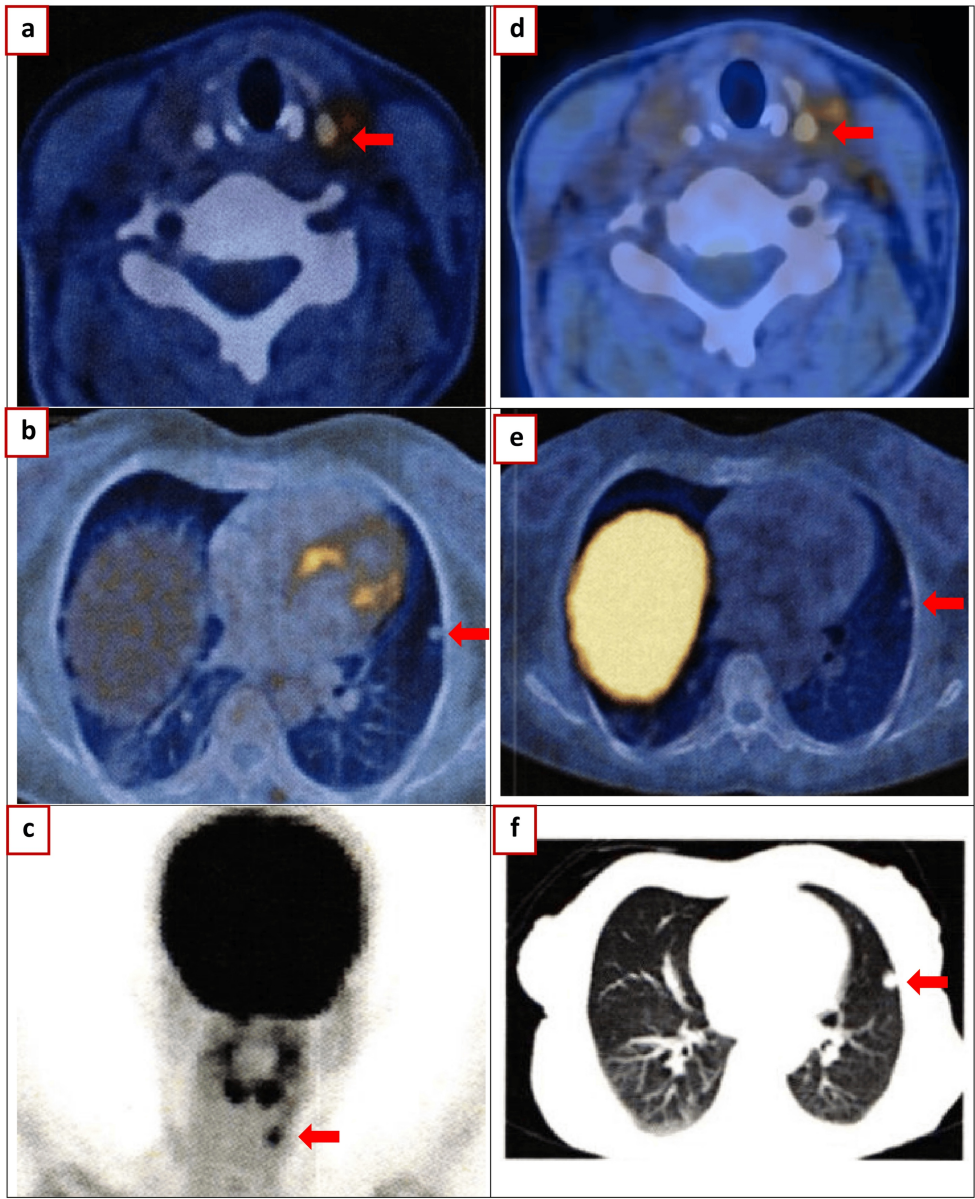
Nuclear medicine imaging (a-c) 2023 August: 18 F-fluorodeoxyglucose (FDG) PET/CT: “New” nodule/lymph node in the left mid jugular region standardized uptake value (SUV): 4.8 (8 × 6 mm). Left lower cervical and supraclavicular lymph nodes SUV: 1.9 (largest 11 × 9 mm). Left lung nodules SUV: 1.6 (7.5 mm). (d,e) 2023 August: Ga68 Dotanoc PET-CT: “New” nodule/lymph node in the left mid jugular region SUV: 3.2 (8 × 6 mm). Left lower cervical and supraclavicular lymph nodes SUV: 1.6 (largest 11 × 9 mm). Left lung nodules SUV: 1.6 (7.5 mm). (f) 2024 May: Chest CT scan showing metastatic left lower lobe lung nodule.
